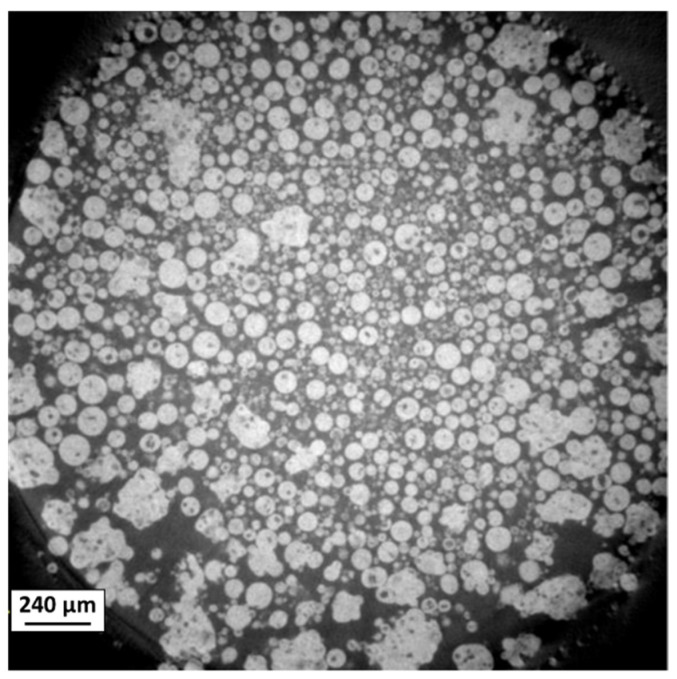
X-ray tomography slide of batch 1 granules.
